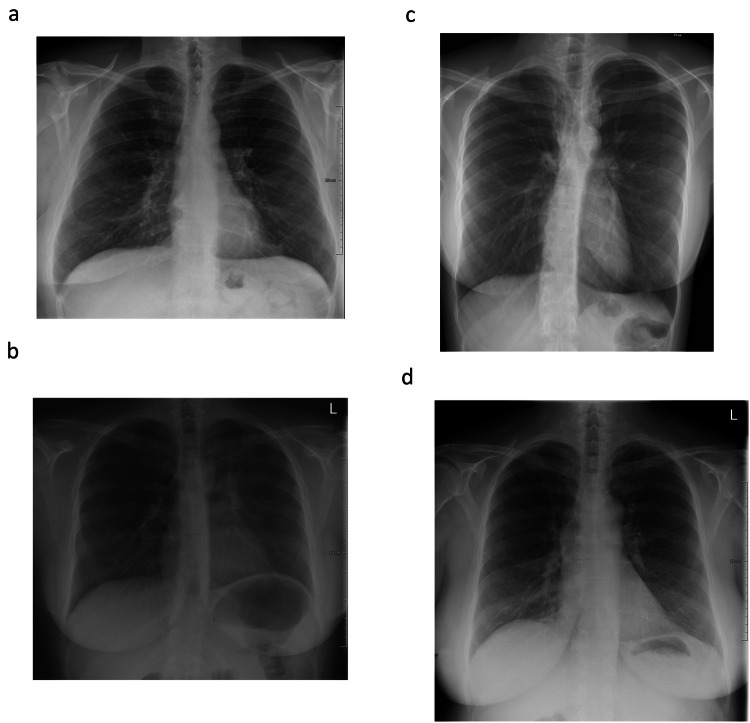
The four cases with urgent findings missed by the AI system at the conservative operating point. a From earlier CT known stable nodule in the right lower quadrant, with characteristics of a subpleural lymph node on CT. Classified as an urgent finding by two of the three radiologists. b CT confirmed left hilar mass. Classified as an urgent finding by all three radiologists. c Suspicion of minor post-tuberculosis fibrotic changes in the upper right lung. Classified as an urgent finding by two of the three radiologists. d In the original report description of small right paracardial infiltrate. Classified as an urgent finding by two of the three radiologists
